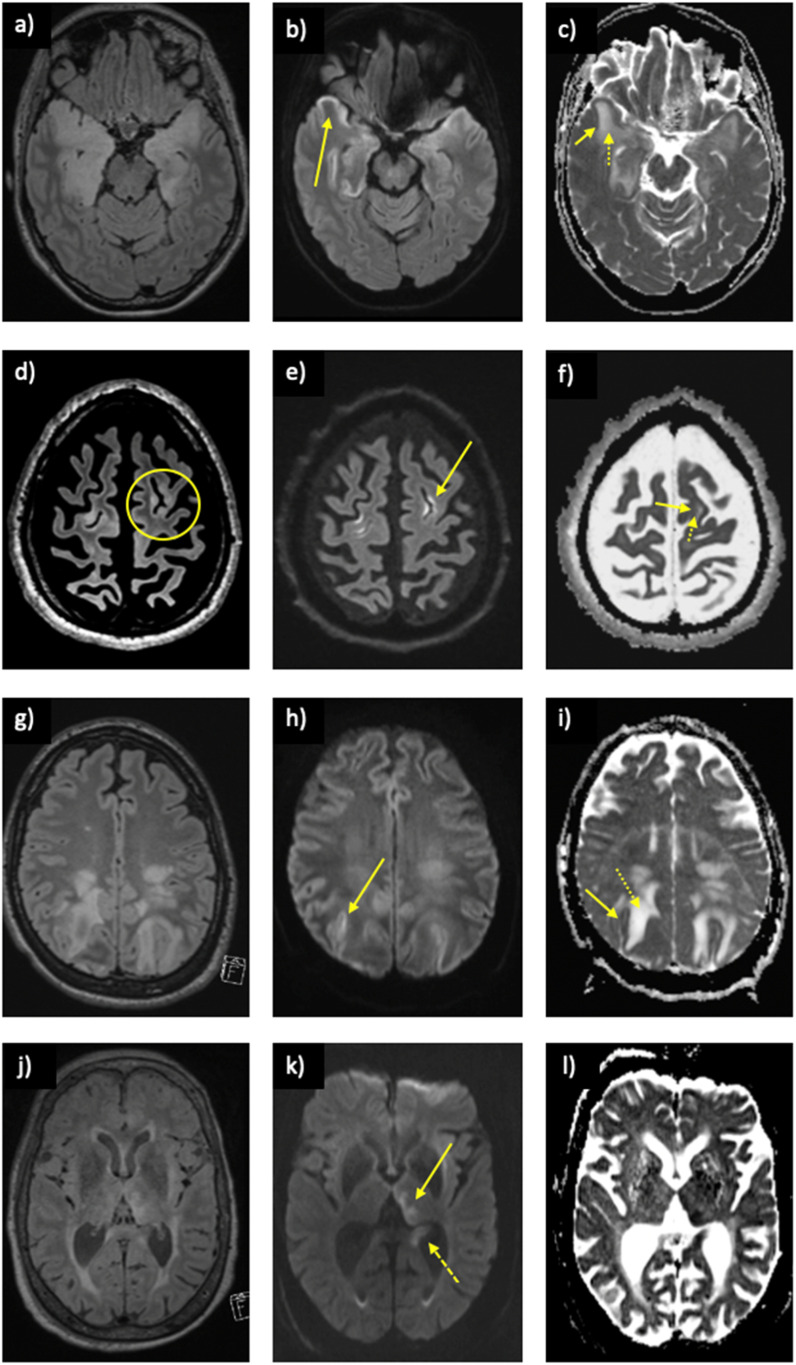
Other causes of encephalitis also demonstrating the split ADC sign. Herpes simplex encephalitis on (a) FLAIR demonstrating signal change within the temporal lobes bilaterally, (b) restricted diffusion on DWI in the cortex (arrow), and (c) the split ADC sign with low cortical ADC (solid arrow) and high subcortical ADC (dotted arrow). MELAS on (d) FLAIR demonstrating signal change within the cortex and subcortical white matter in the left frontal lobe (circle), (e) restricted diffusion on DWI in the cortex (arrow), and (f) the split ADC sign with low cortical ADC (solid arrow) and high subcortical ADC (dotted arrow). PRES on (g) FLAIR demonstrating signal change within the parietal lobes bilaterally, (h) restricted diffusion on DWI in the cortex (arrow), and (i) the split ADC sign with low cortical ADC (solid arrow) and high subcortical ADC (dotted arrow). Peri-ictal setting with a positive split ADC sign with concurrent evidence of a recent seizure including (j) FLAIR demonstrating signal change within the left pulvinar and hippocampus, (k) restricted diffusion on DWI in the pulvinar (solid arrow), and hippocampus (dashed arrow) with corresponding low ADC on (i).
